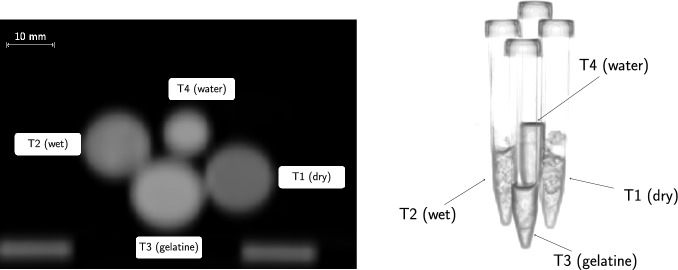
Top view of a CT slice (right) and 3D rendering of the CT (left) of the setup with the four tubes taped together
